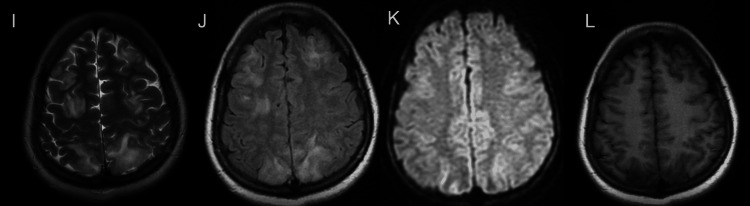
MRI at the level of centrum semiovale. Altered signal intensity which appears hyperintense on T2WI (I), FLAIR (J); T2 shine through in DWI (K); hypointense on T1WI (L) involving bilateral posterior parietal and high frontal regions. DWI - Diffusion-weighted imaging FLAIR - Fluid attenuated inversion recovery MRI - Magnetic resonance imaging T1WI - T1 weighted imaging T2WI - T2 weighted imaging
